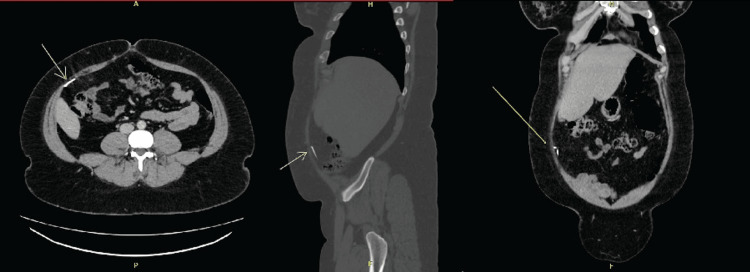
Computed tomography scan of the abdomen and pelvis with contrast with the intra-abdominal foreign body denoted by a yellow arrow in transverse, sagittal, and coronal sections
